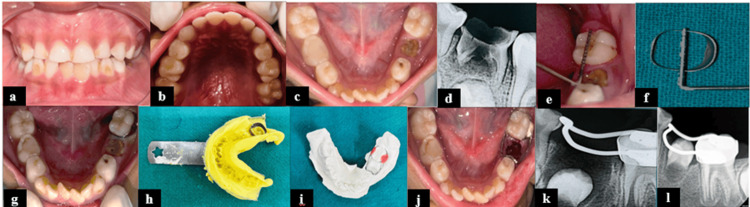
Preformed band and custom-made loop space maintainer for Case 1. (a) Intraoral frontal view; (b) intraoral preoperative maxillary occlusal view; (c) intraoral preoperative mandibular occlusal view showing root stump in relation to 75 and dental caries with 74; (d) radiograph showing crown destruction and root resorption involving furcation in relation to 75; (e) assessing the mesiodistal diameter of tooth 36; (f) assessing the mesiodistal diameter of the band; (g) assessment of band fit; (h) alginate impression with band; (i) working model with band and loop; (j) band and loop cementation with respect to 75; (k) radiograph showing band and loop with respect to 75; and (l) follow-up radiograph at six months.
